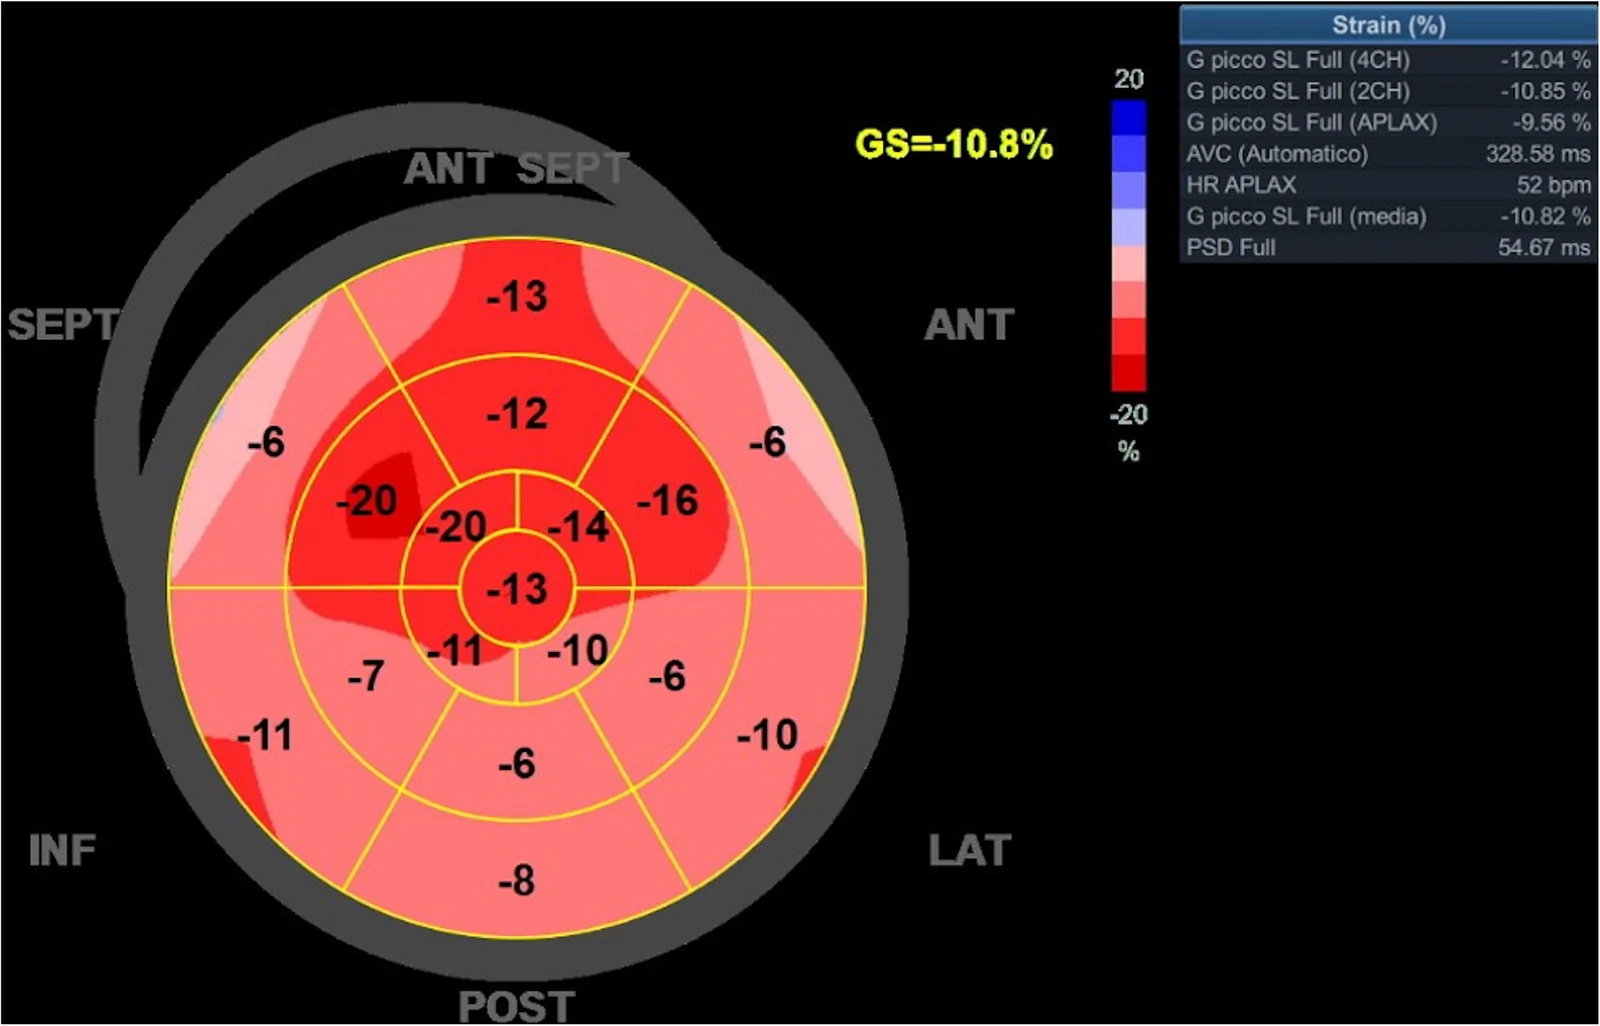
Transthoracic Echocardiography Demonstrated Preserved Left Ventricular Ejection Fraction With Increased Wall Thickness and Biatrial Enlargement Global longitudinal strain (GLS) analysis revealed severely reduced myocardial deformation (−10.8%) with relative preservation of apical segments, producing a characteristic “apical sparing” pattern. This strain distribution is highly suggestive of cardiac amyloidosis and represents an important noninvasive clue in patients with heart failure with preserved ejection fraction and disproportionate biomarker elevation.
